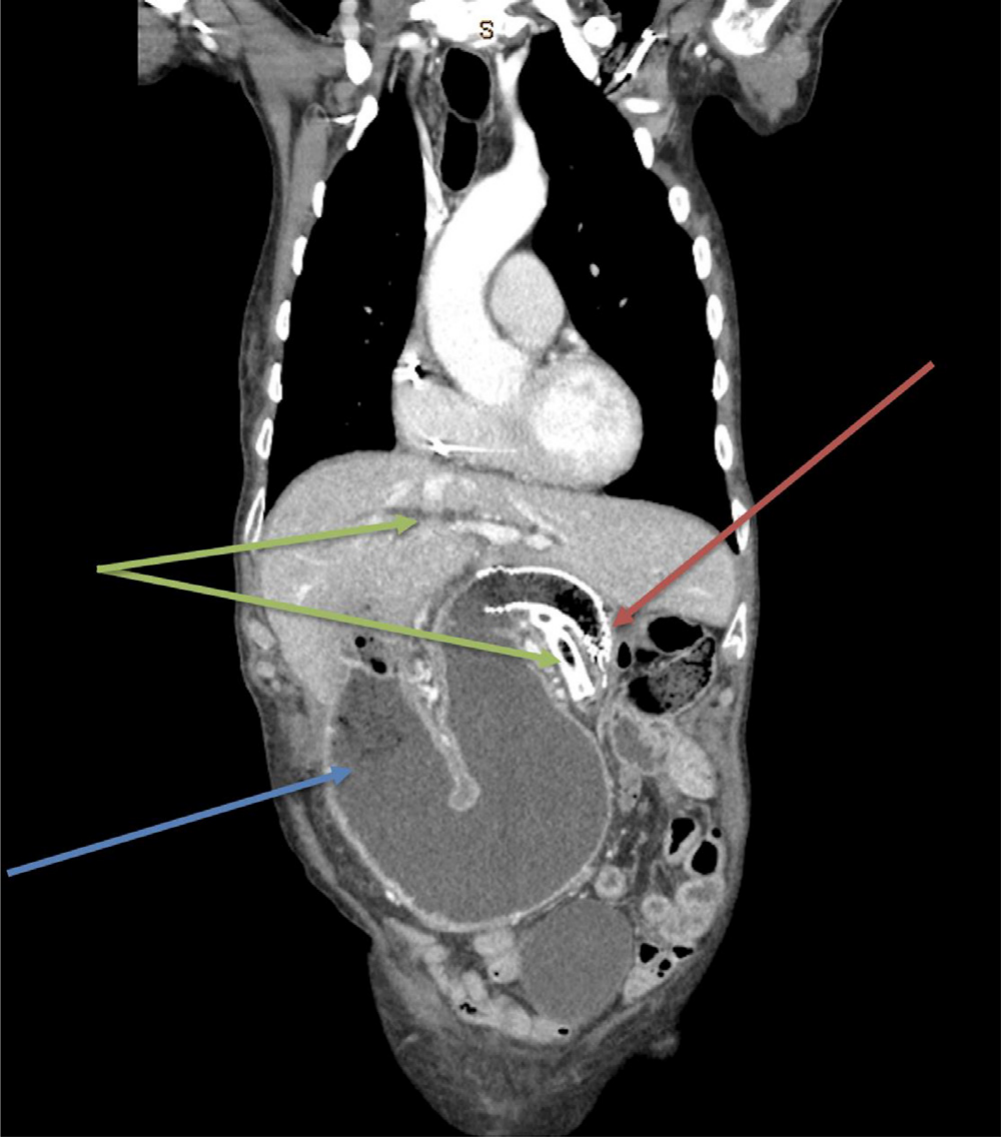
Coronal slice of CT chest, abdomen, and pelvis with contrast demonstrating distended right-sided stomach and midline liver, with duodenal and biliary stenting in-situ. Red arrow – Compression of the distal aspect of the duodenal stent. Blue arrow – Distension of the right-sided stomach to the level of the duodenal stent. Green arrows – Biliary stent in situ with intrahepatic biliary dilatation and lack of pneumobillia.
